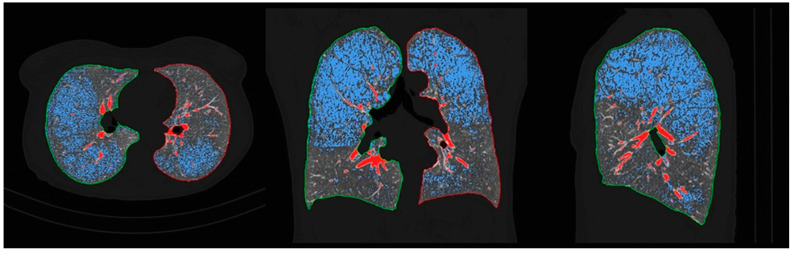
Axial, coronal, and sagittal chest CT scan with postprocessing in Syngo.Pulmo3D. Blue zones represent low attenuation areas/volumes (LAV%) indicating emphysema.
